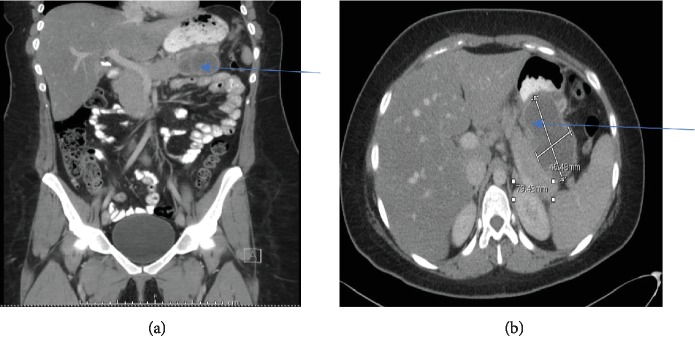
CT of the abdomen and pelvis with oral and IV contrast showing a large multilobulated upper abdominal mass (arrow) inseparable from the body and tail of the pancreas (a). The tumor (10 cm × 9 cm × 7 cm) filled the lesser sac (arrow) and wrapped around the gastric fundus (b).
